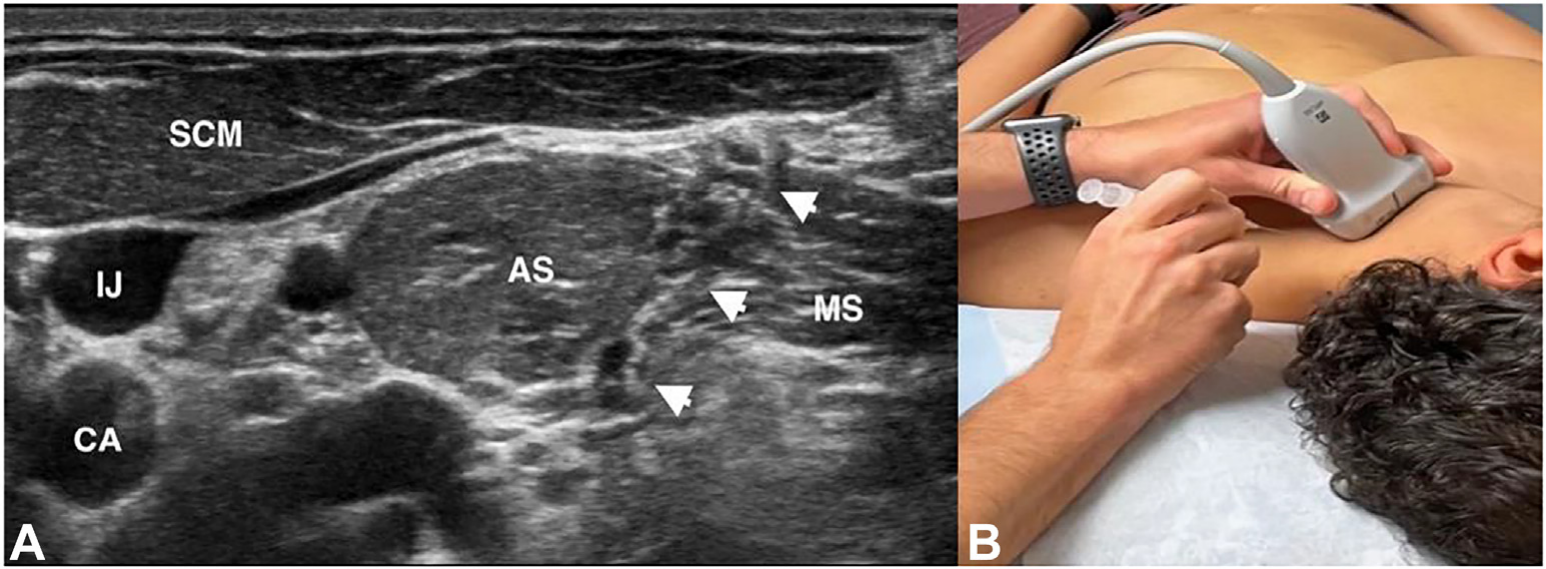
Ultrasound-guided injection of local anesthetic targeted to the scalene triangle. The white arrows indicate the needle. AS, anterior scalene; CA, carotid artery; IJ, internal jugular vein; MS, middle scalene; SCM, sternocleidomastoid.
